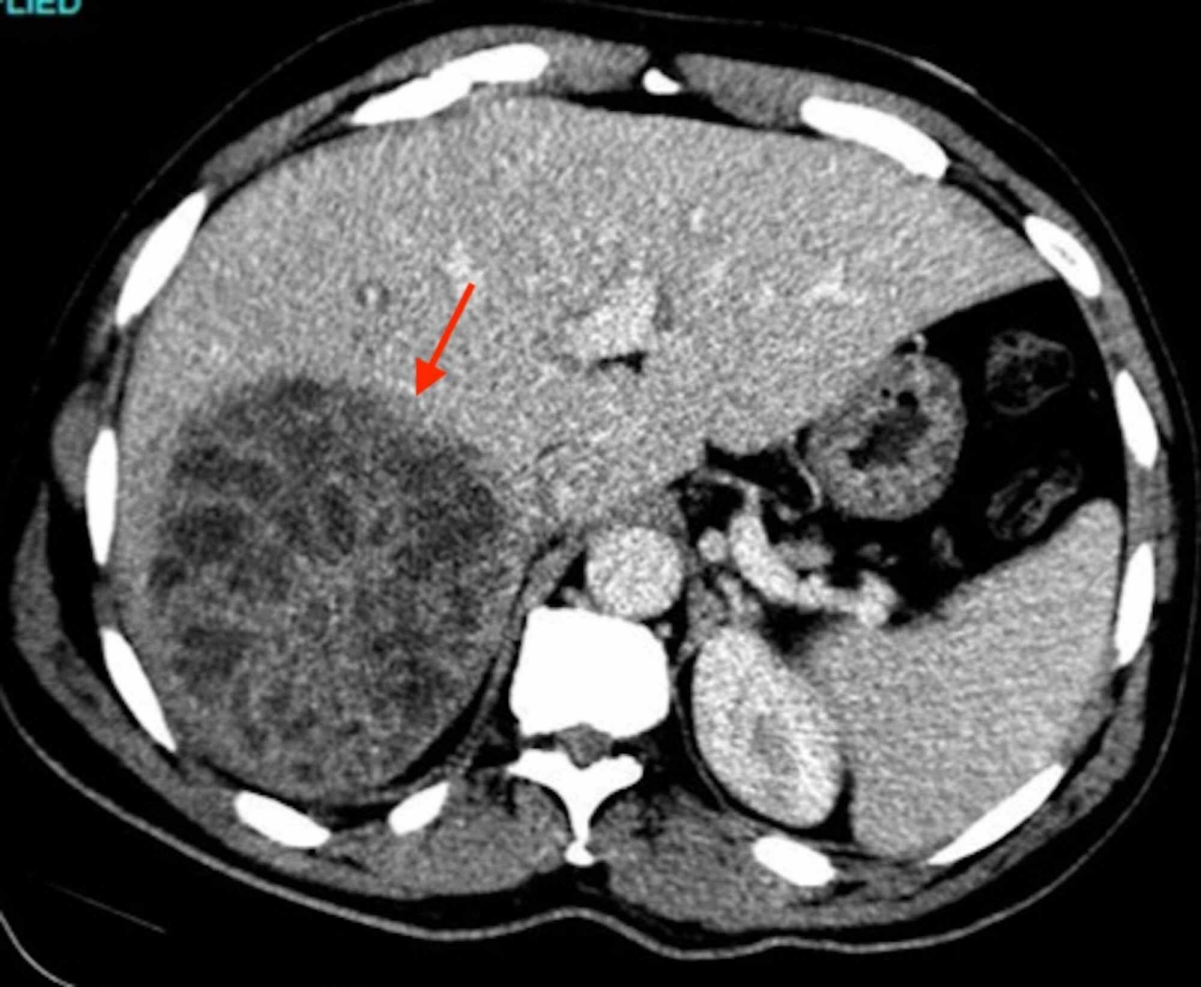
CT of the abdomen demonstrating a large 10 cm heterogeneous hypodensity in the post right hepatic lobe likely a liver abscess. The arrow points to area of interest.
